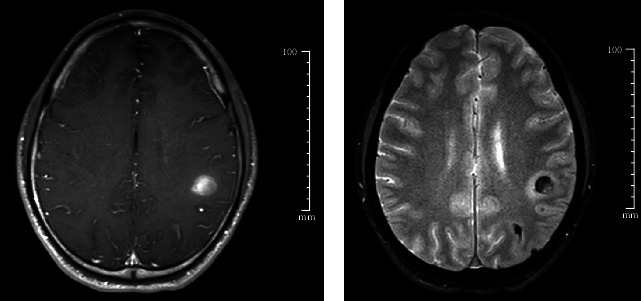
Brain magnetic resonance imaging with contrast. A circumscribed lesion with ring enhancement and hyperintensity on the T1-weighted image (a) with surrounding edema visible on the T2-weighted image (b).
